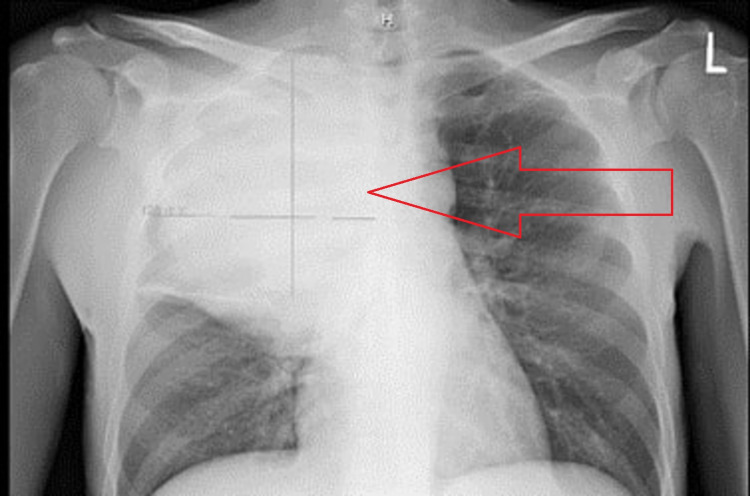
CXR shows a uniform opaque mass occupying the right upper hemithorax of around 16 x 13 cm in size. CXR, chest X-ray
